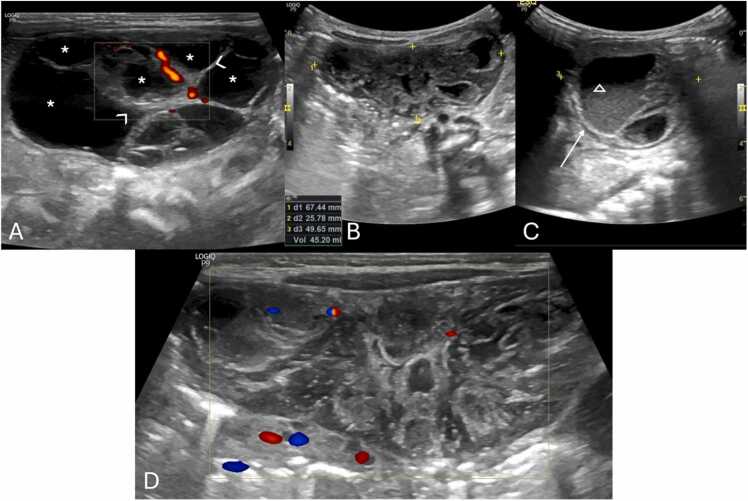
(A–C) Pelvic Ultrasound of a 2-day-old newborn with prenatal diagnosis of pelvic intra-abdominal cystic formations, likely of adnexal origin. The right ovary, in the transverse plane with Power Doppler (A) is show, and is remarkably enlarged, containing multiple central cysts (* in A), separated by septa (arrowheads in A) and preserved vascular flow. Transverse and sagittal (B and C, respectively) pelvic US image depicts the left ovary as a large (volume of 45 cc), complex cystic mass (between calipers in B and C), predominantly solid, containing cystic areas, some with fluid-debris levels (triangle in C). Note the presence of the double wall sign (arrow in C), suggesting infarction. Transverse color-Doppler image (D) of the left ovary shown in (B and C) with general decreased vascular flow. Given the location and imaging findings the diagnosis of left ovarian torsion was suggested, and an exploratory laparoscopy was performed, confirming the diagnosis of left ovarian torsion (no torsion was noted on the right ovary and the cysts were aspirated).
